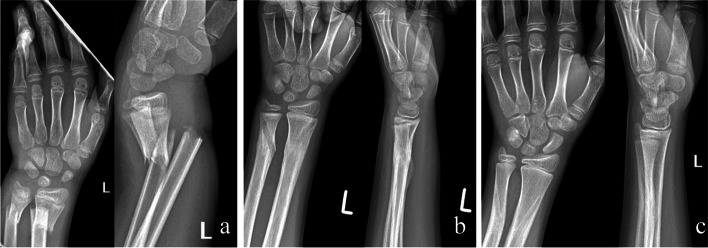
Distal forearm fracture of a 11-years old boy treated with Activa Pin™ a: shortened and displaced unstable distal meta-diaphyseal fracture b: X-rays made after 12 weeks of surgery c: X-rays made 24 months after surgery
